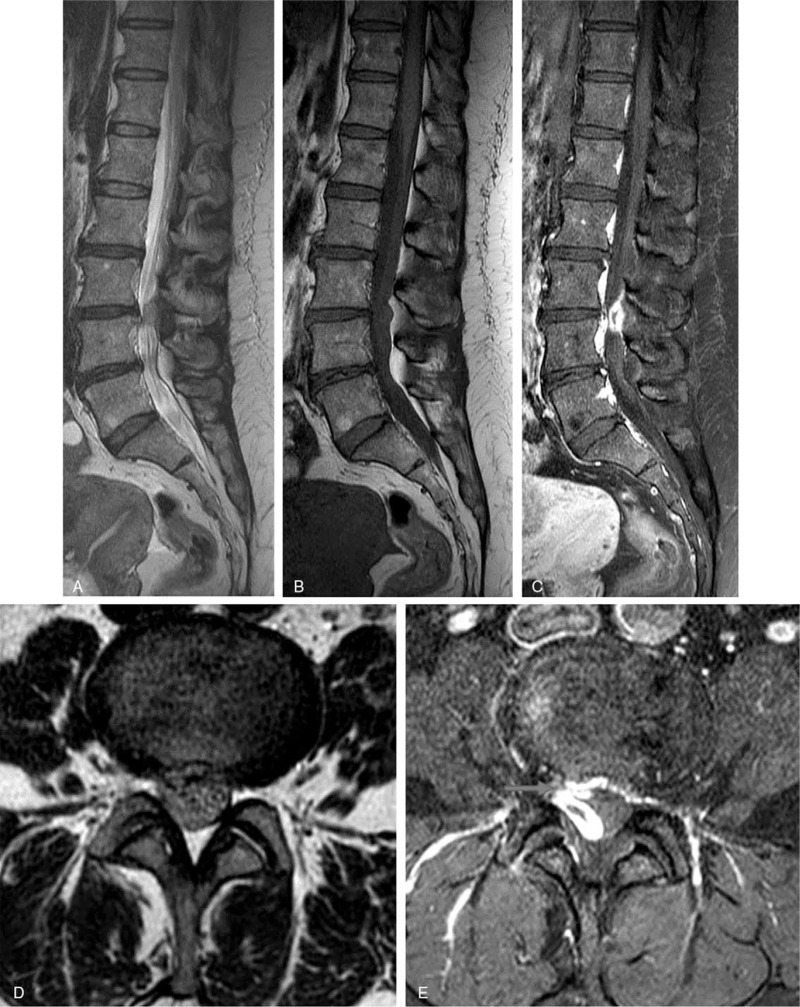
Preoperative MRI of the lumbar spine without and with contrast enhancement. Sagittal T2-weighted MRI (A) and sagittal (B) and axial T1-weighted MRI (D) showed a posterolateral epidural mass compressing the thecal sac. Enhanced T1-weighted MRI in the sagittal and axial planes showed a lesion with heterogenous ring-like enhancement at the L3-L4 level and demonstrated a sequestrated fragment with posterior migration (C and E). On the axial images, the route of migration in the form of a tract-like enhancement extending from the outer aspect of the disc to the posterolateral epidural space was found (Red arrow).
